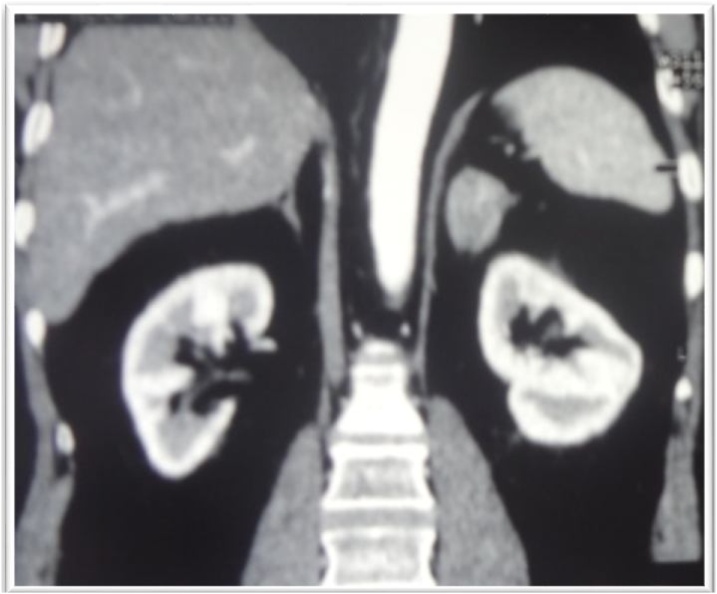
CT urogram showing left adrenal mass (case 1).
